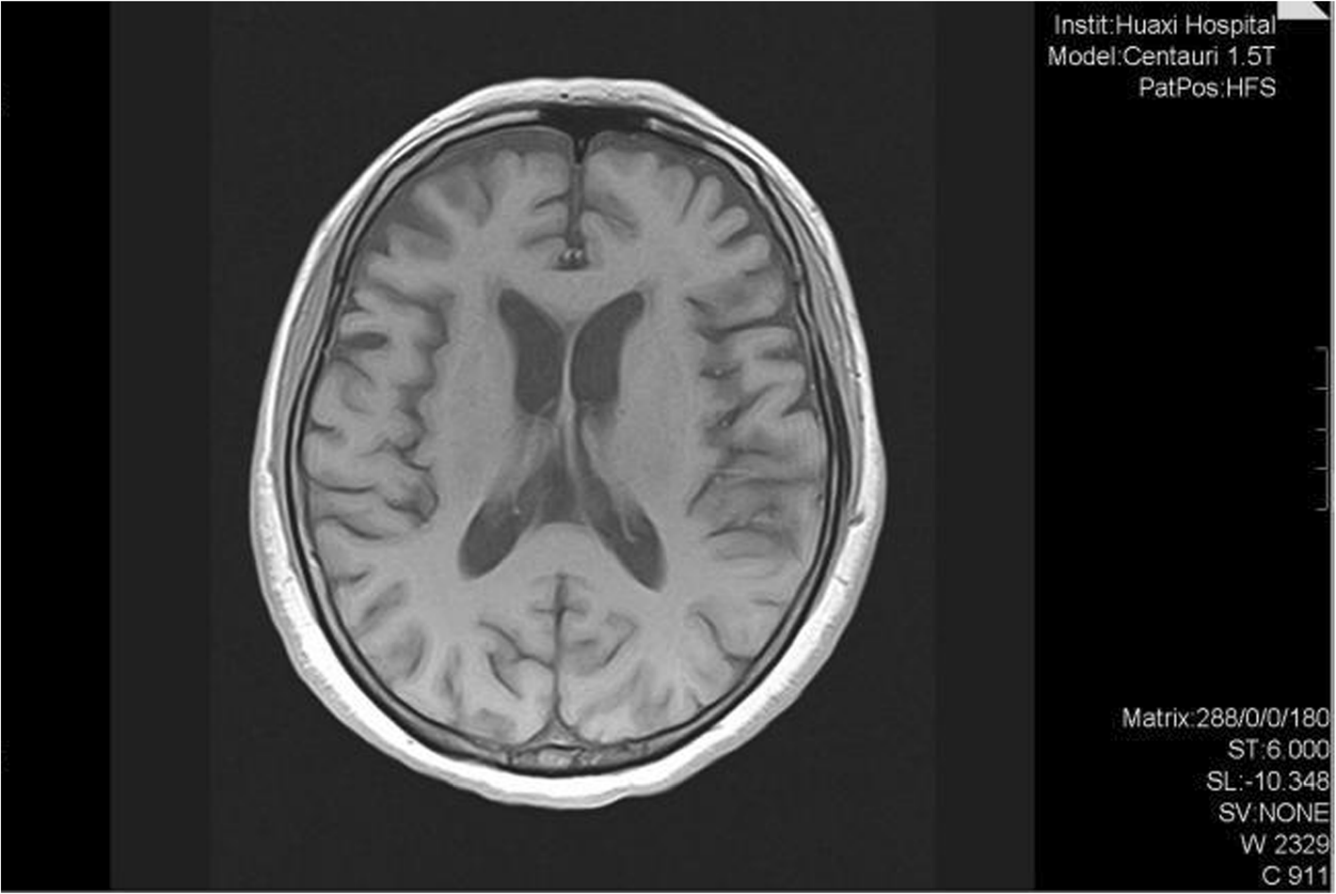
The brain MRI in August 2019. Brain MRI Showed moderate bilateral frontotemporal atrophy
